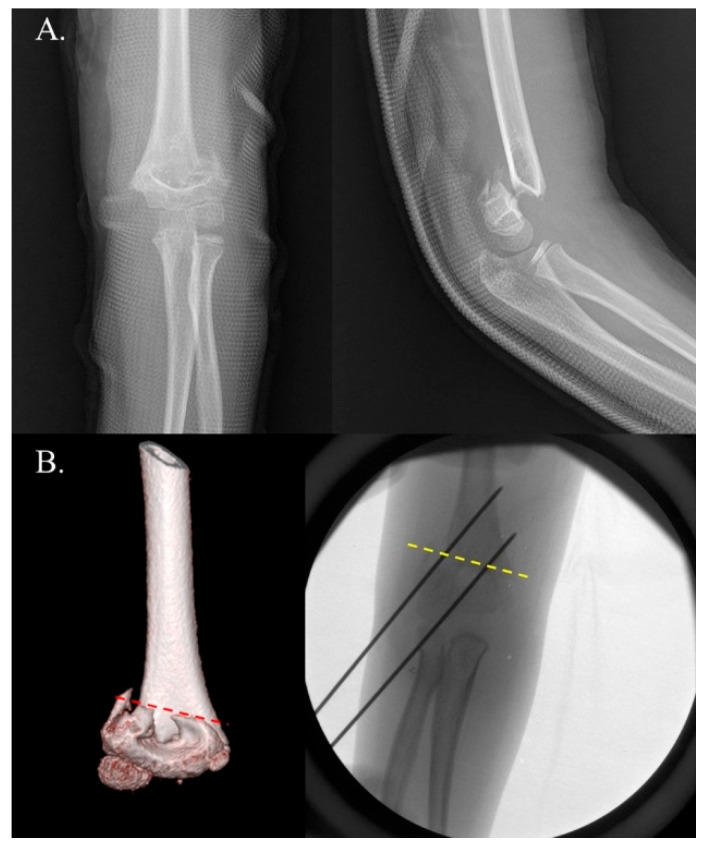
Example of a high oblique fracture on the radial side in a 7-year-old boy with a Gartland type III supracondylar fracture. Panel (A) displays the Gartland type III fracture in preoperative radiographs. Panel (B) demonstrates that this pattern (red and yellow dashed lines) can be confirmed on preoperative CT scans and C-arm images taken intraoperatively.
